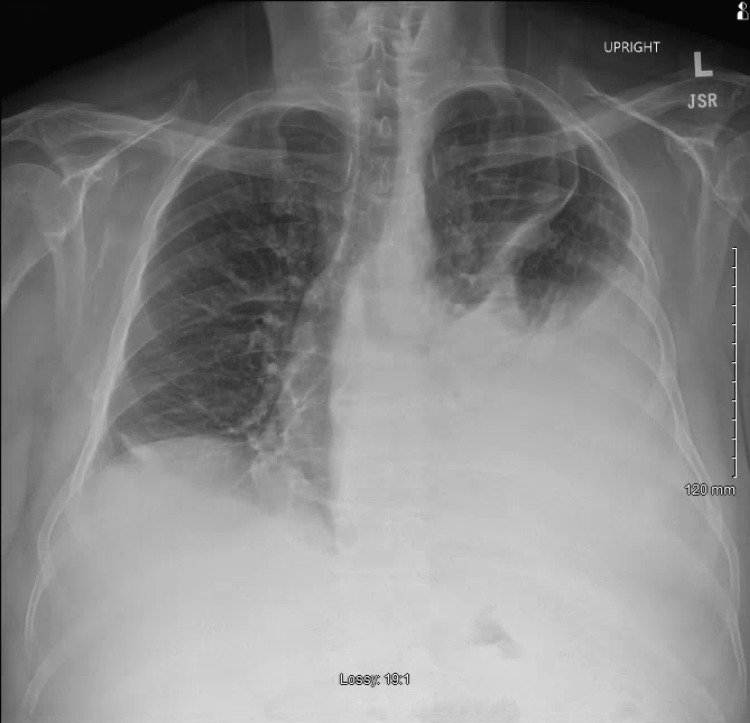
Anterior-posterior chest radiograph demonstrating a large left-sided pleural effusion and small right-sided pleural effusion.
